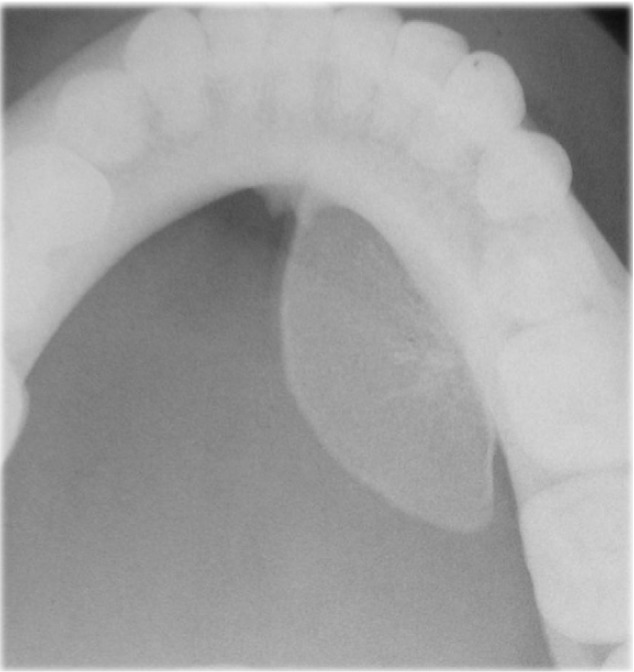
Occlusal radiograph revealed a well defined lesion surrounded by a sclerotic margin, containing reactive bone spicules which are characteristic for intraosseous cavernous hemangioma.
